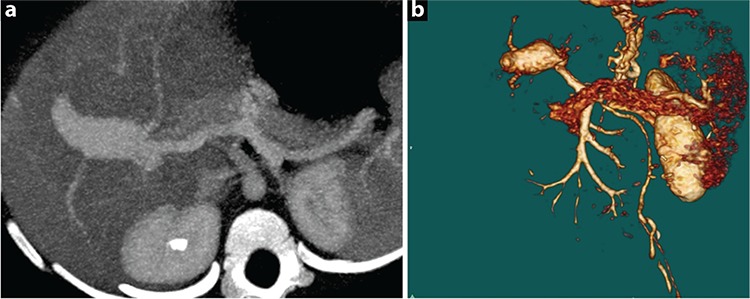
a, b. Preoperative maximal inspiratory pressure (a) and 3D reconstructed dynamic abdominal computed tomography images showing fusiform aneurysmal dilation in the proximal end of the intrahepatic portal vein and prominent esophageal varices (b).
